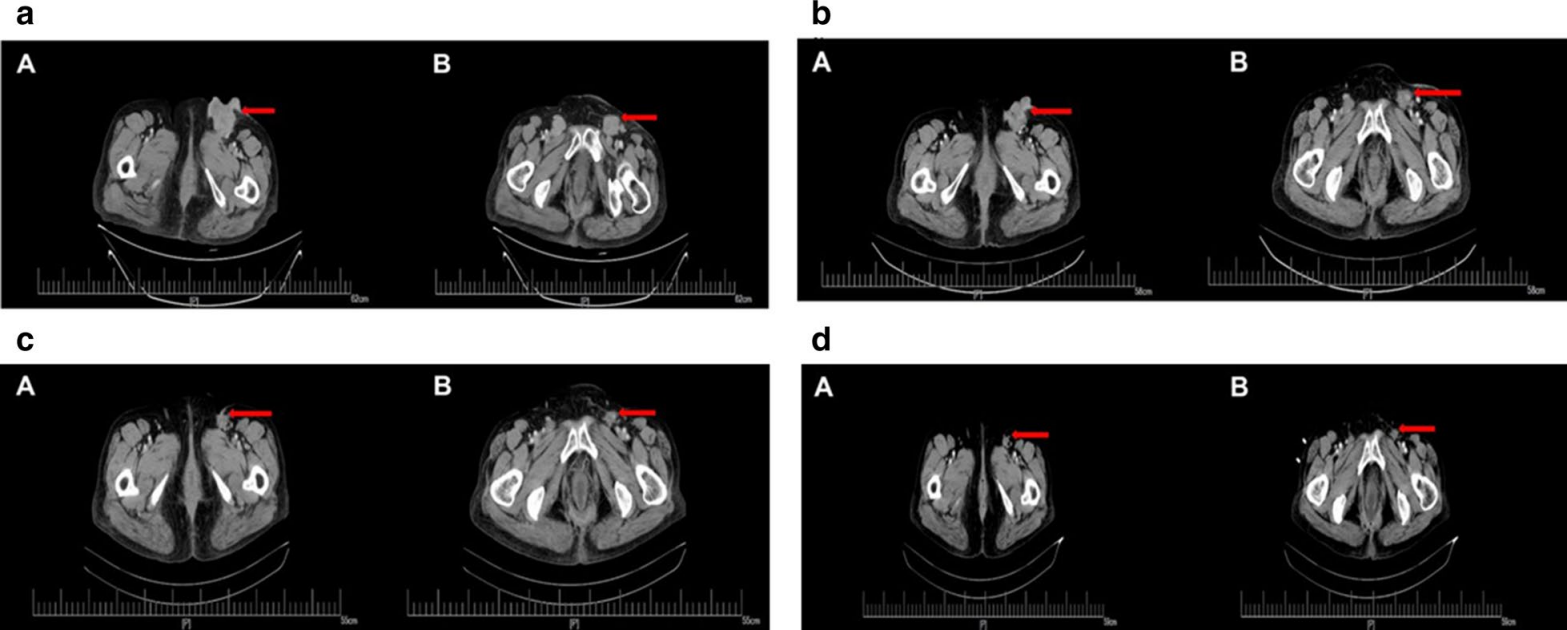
CT: pelvic cavity to assess disease status and progression. CT image of pelvic cavity to assess disease status before starting treatment with regorafenib and sintilimab (a Arrows indicate metastatic focus before treatment). CT image of pelvic cavity to assess disease progression with regorafenib and sintilimab treatment before treatment (b–d Arrows indicate metastatic focus shows that the tumor size has decreased during treatment duration)
